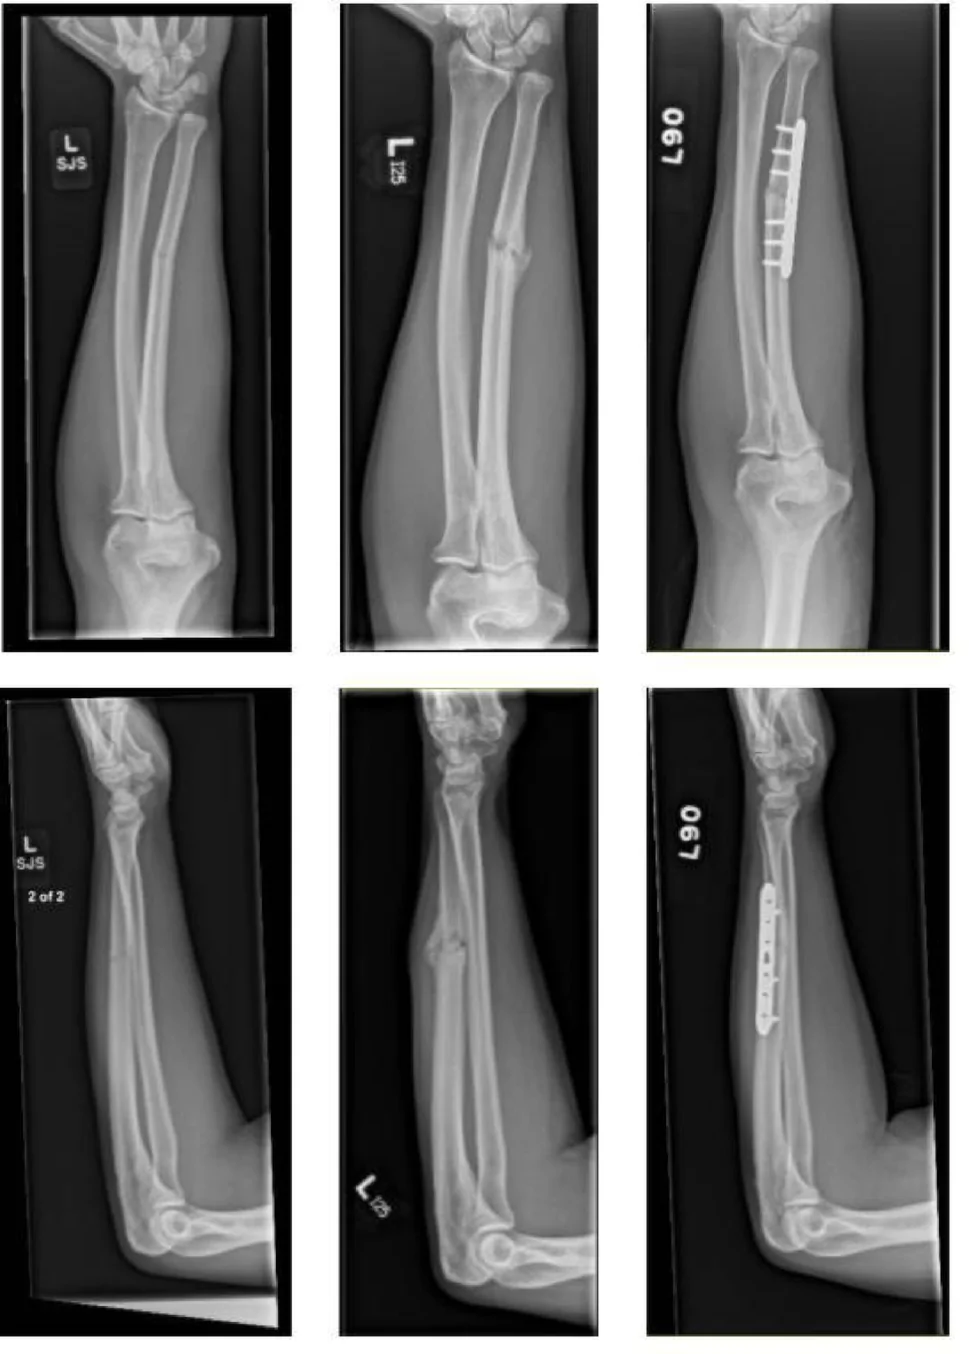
Anteroposterior and lateral forearm radiographs from a patient with a nonoperatively managed distal ulna fracture (left panel) that progressed to nonunion (middle panel) and later healed well following open reduction internal fixation (right panel)
